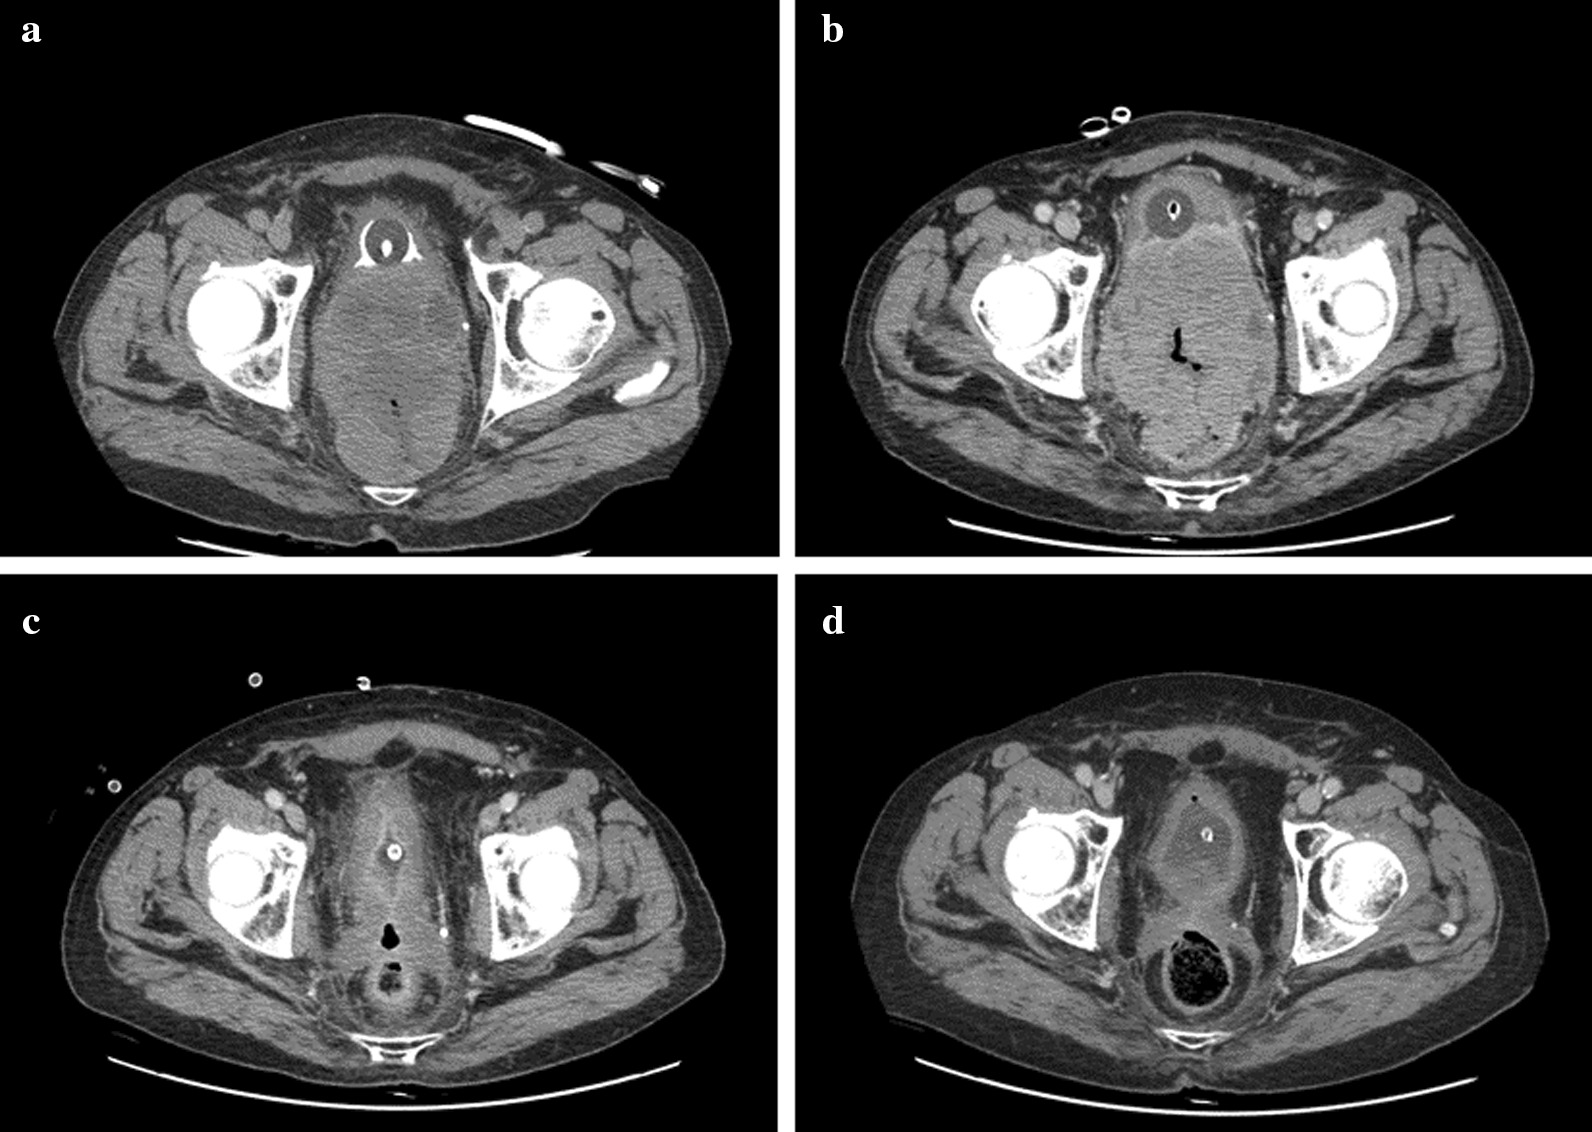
Radiographic CT response to treatment of local disease. a CT images of advanced disease at staging. b CT images after chemotherapy and LH RH analogue. c CT images of radiation response after two months. d CT images 9 months after radiation therapy
